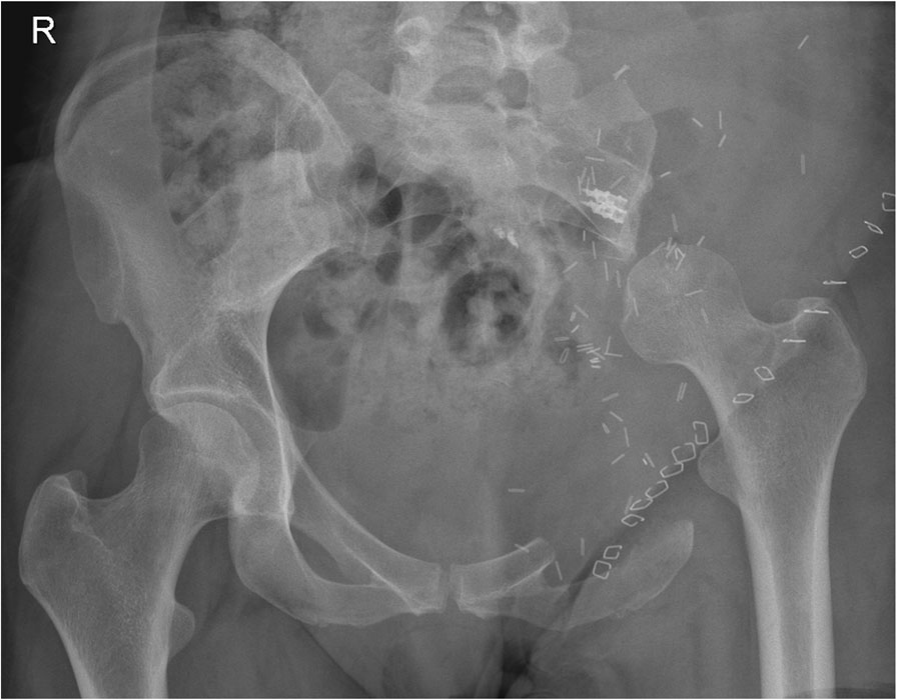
Plain radiograph of a pelvis a.p. after intraarticular, internal hemipelvectomy (types 1–2 and partially 3) and hip transposition. Two screw anchors were implanted in the sacrum for suspension of the femoral head inside an attachment tube
